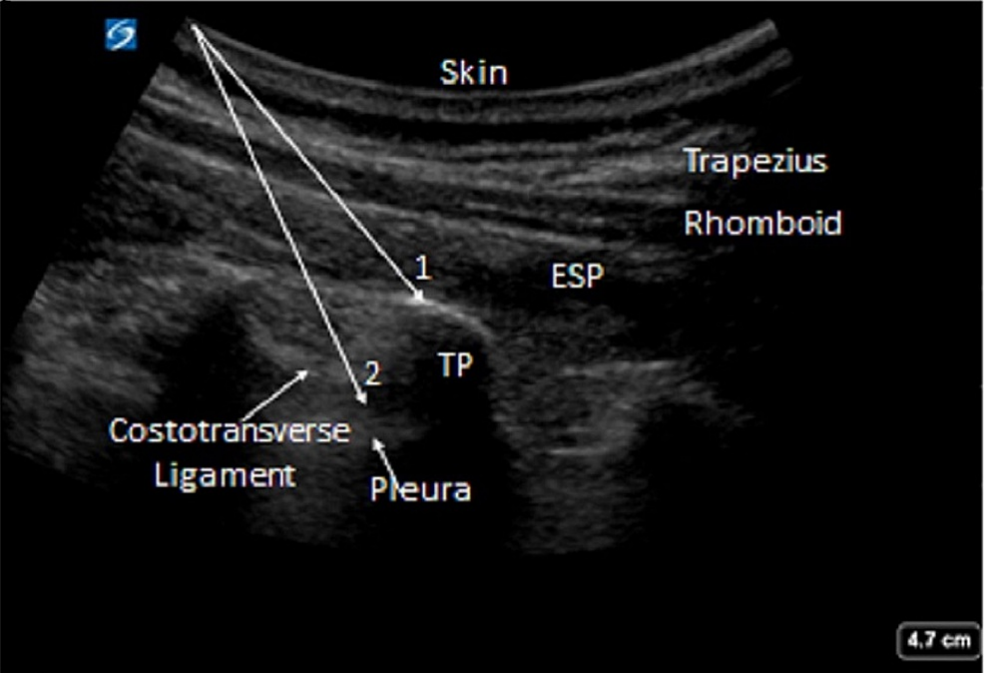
Ultrasound guided ESP (1) and PVB (2) blocks (arrows depict in-plane needle trajectory) ESP: erector spinae plane, PVB: paravertebral block
